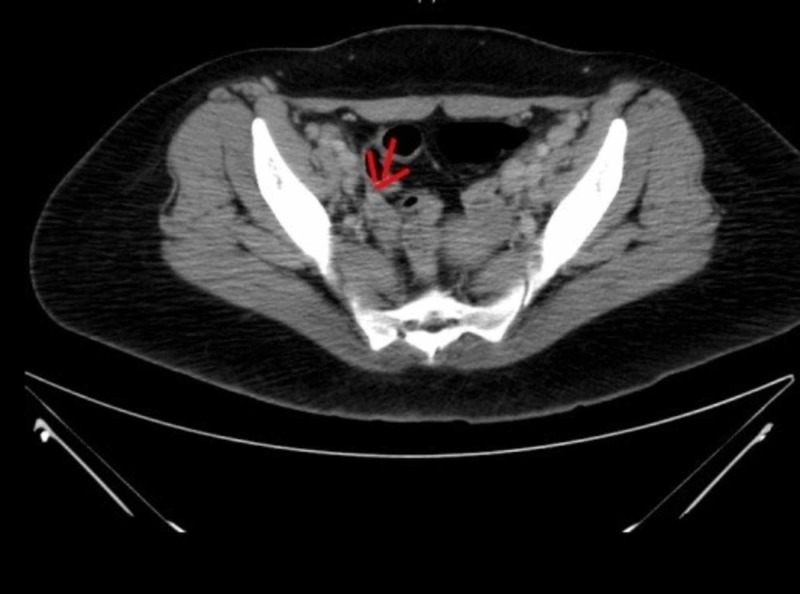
Axial CT scan of the pelvis showed pathologically enlarged inguinal lymph nodes.
